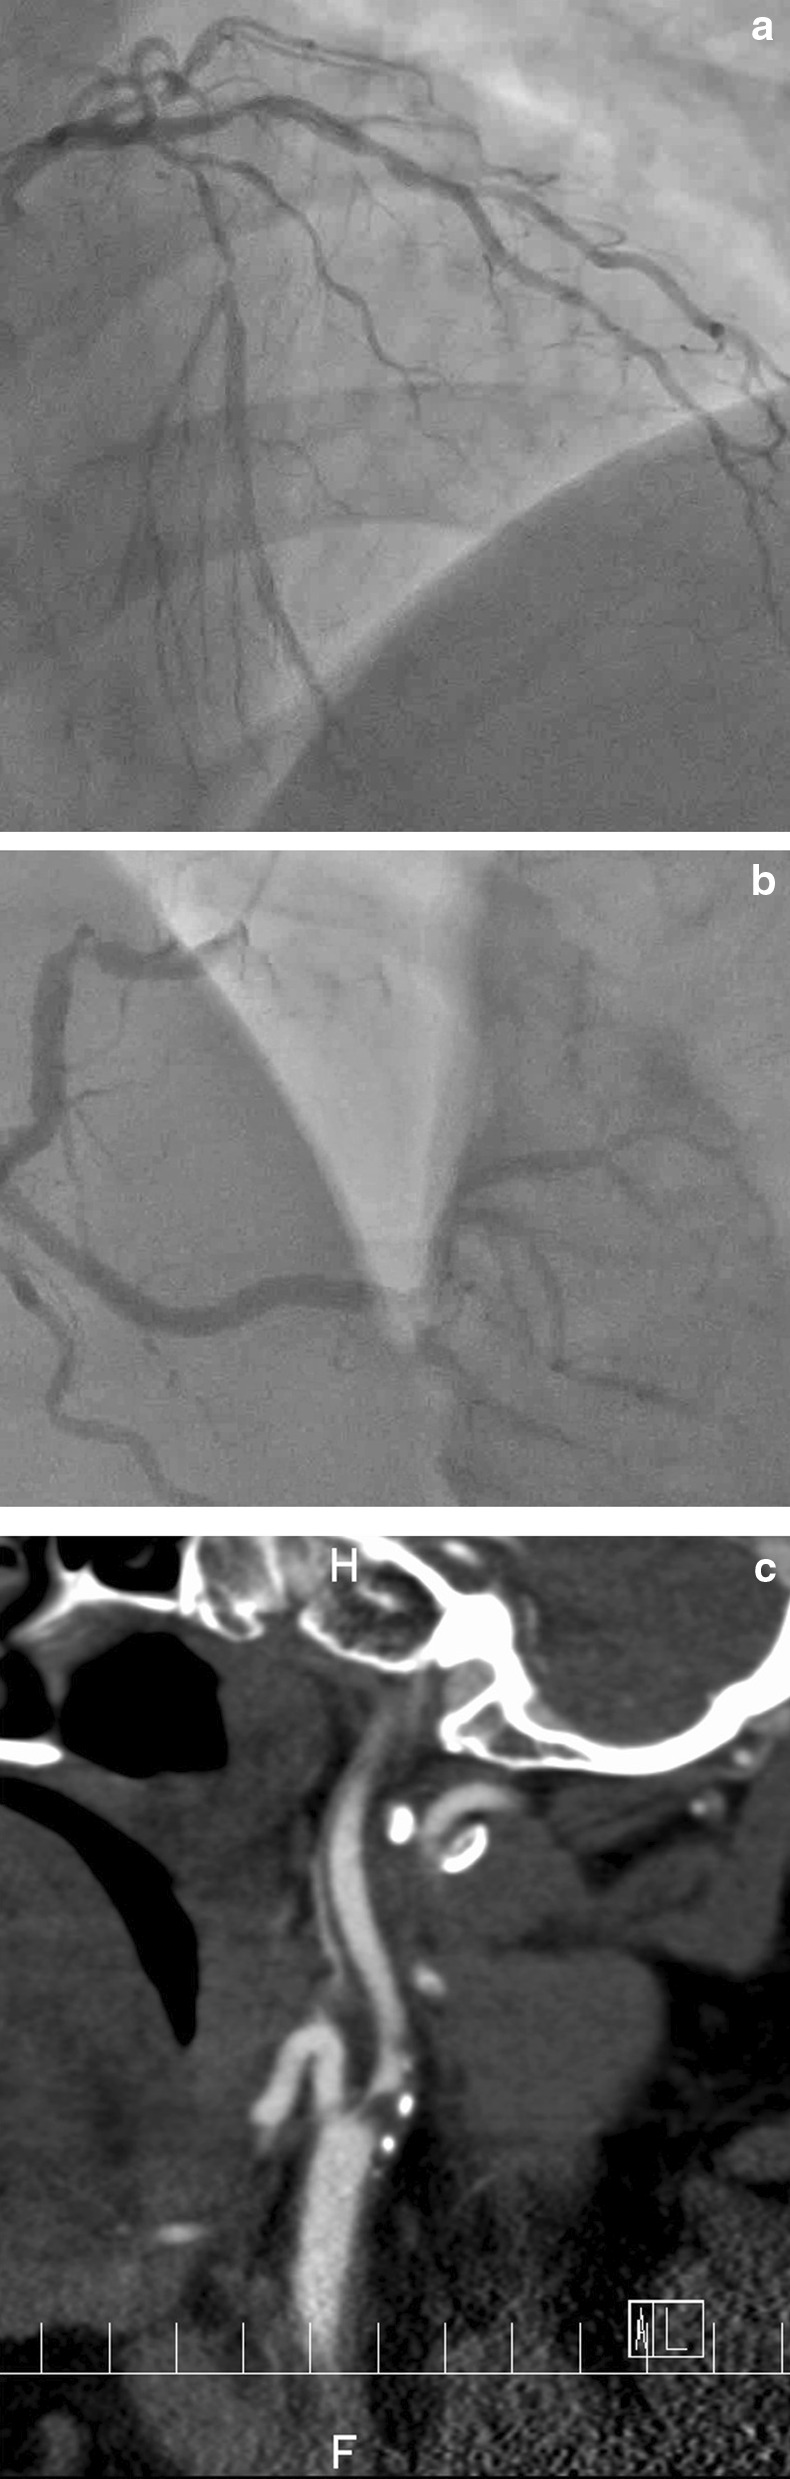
a Preoperative left coronary angiography showing severe stenosis of the left circumflex and left anterior descending arteries. b Preoperative right coronary angiography showing severe stenosis of the right coronary artery. c Preoperative computed tomography scan showing severe stenosis of the left common carotid artery (white arrowhead)
